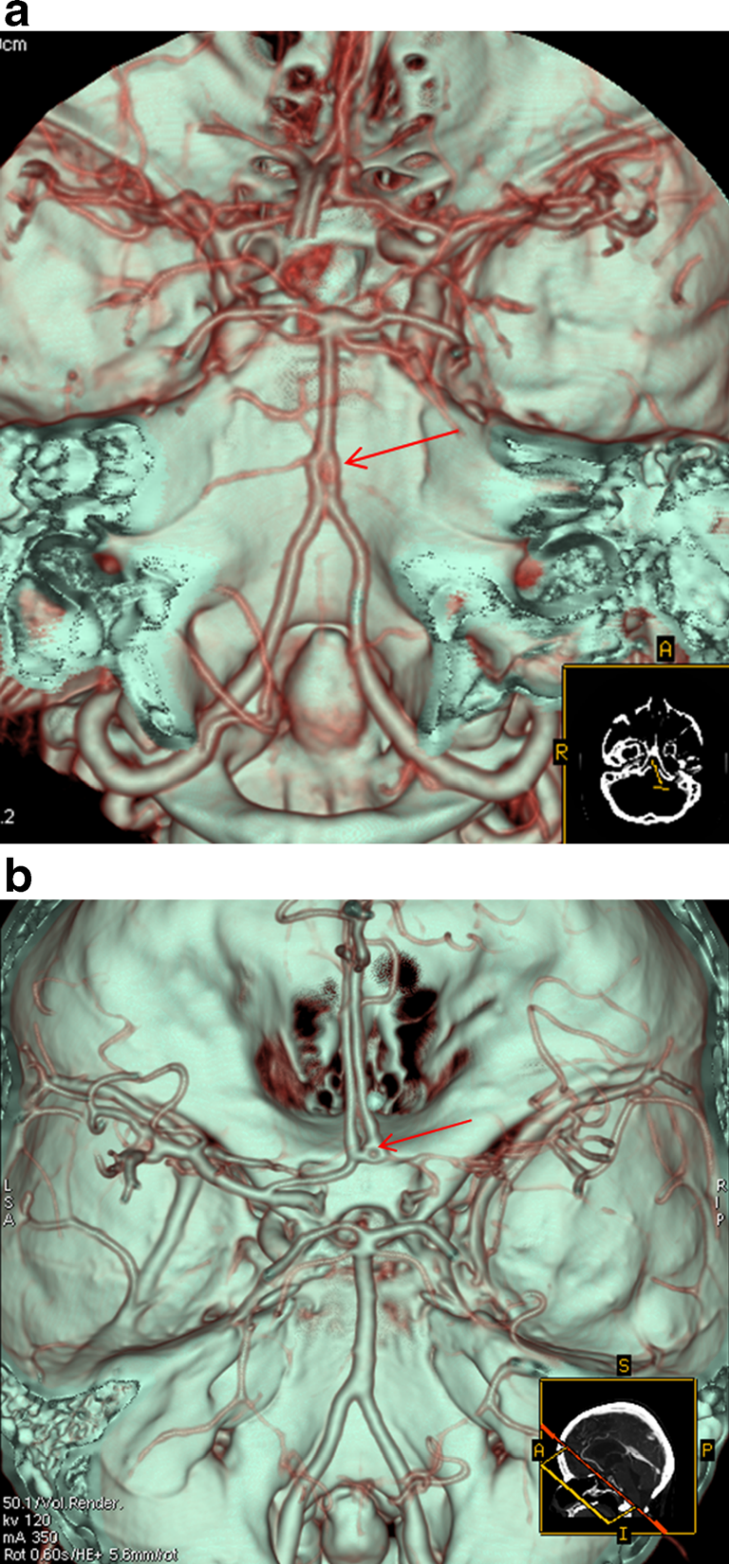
a CTA (VRT 3D reconstructions) shows a proximal basilar artery fenestration (*red arrow*). b CTA (VRT 3D reconstructions) shows an Acom artery fenestration (*red arrow*)
